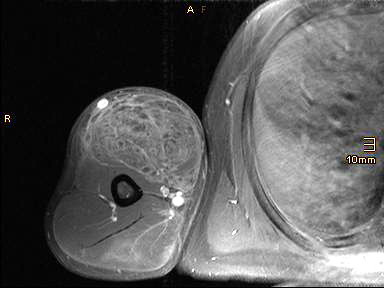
Gadolinium-enhanced transverse T1-spin echo magnetic resonance image sequence with fat saturation of the right arm. The inhomogeneous contrast enhancement of the biceps muscle indicates the presence of inflammation.
